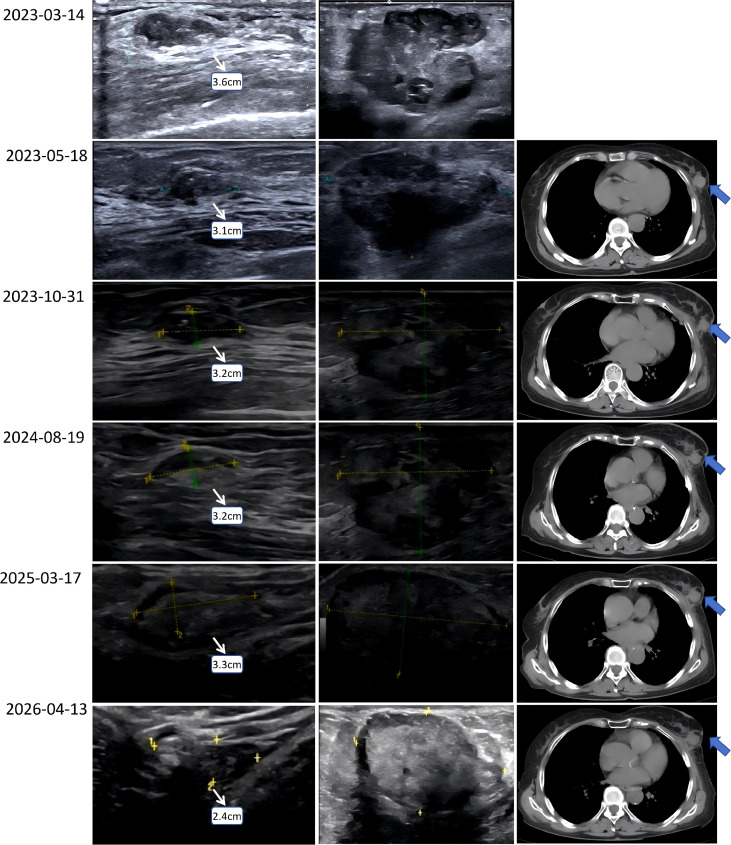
Imaging during the treatment course. Each corresponding row represents follow-up assessments at baseline, 2 months, 6 months, 1 year, 2 years, and 3 years after treatment, including breast ultrasonography and chest CT. The first column shows post-treatment changes in the right breast, left breast, and chest CT of the breast lesions.
